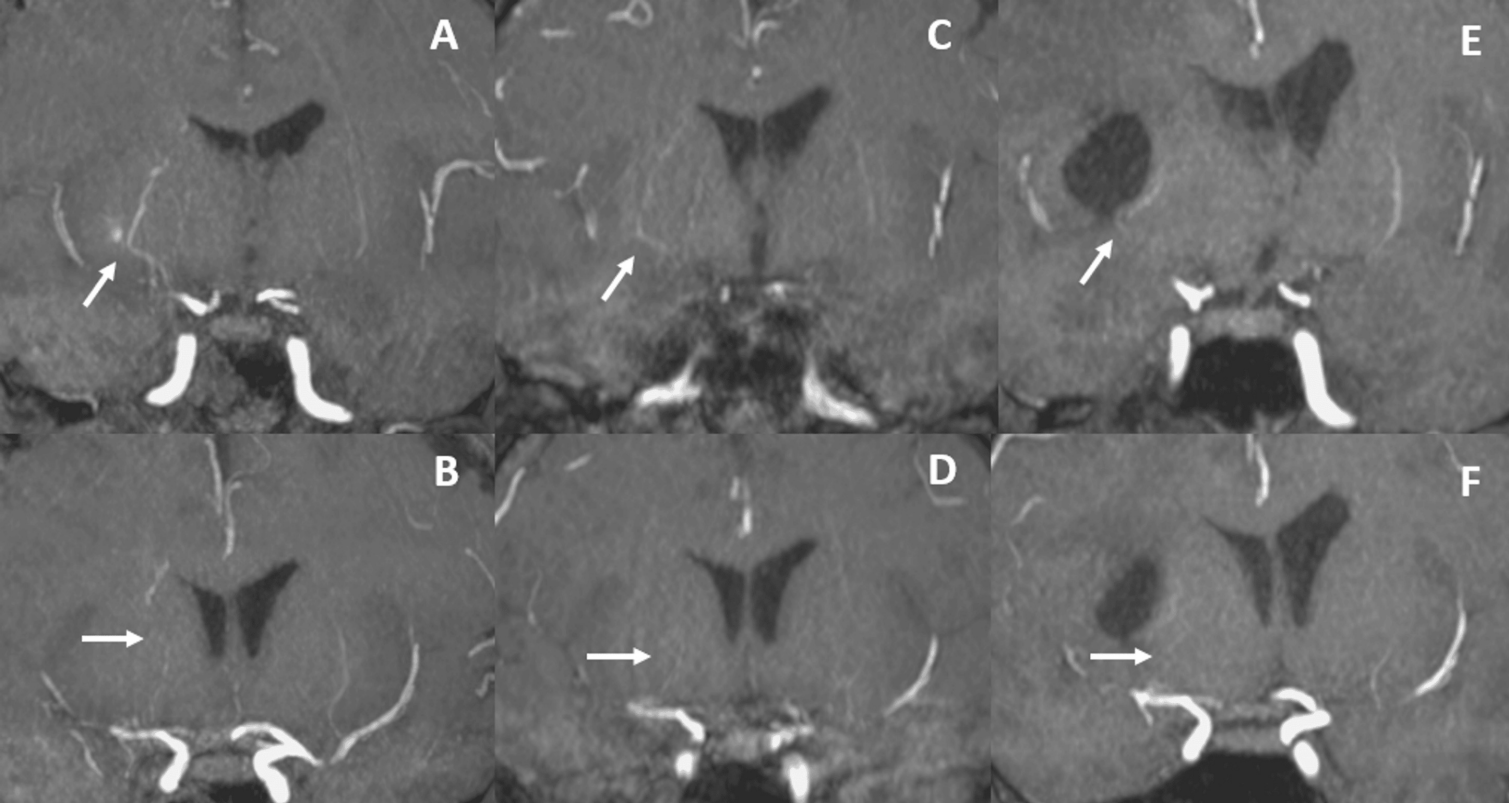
Comparison of MRI, 1 mm slice of Maximum Intensity Projection (MIP) before and after bypass surgery (A, B: Magnetic resonance angiography (MRA) before bypass surgery C, D: MRA seven days after bypass surgery E, F: MRA seven months after bypass surgery). MRI shows shrinkage of periventricular anastomosis (arrow).
